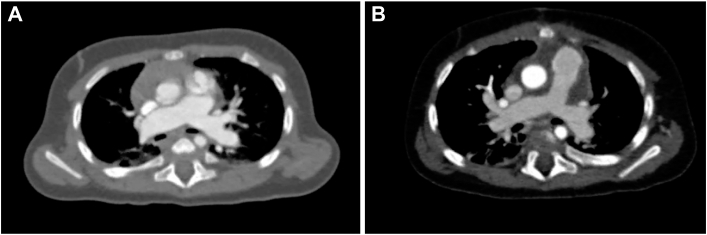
(A) Preoperative computed tomographic scan shows severe bronchial compression due to dilated central pulmonary arteries. (B) Postoperative computed tomographic scan demonstrates significant resolution of bronchial compression after transannular repair with a half-skeletonized handmade valved conduit.
